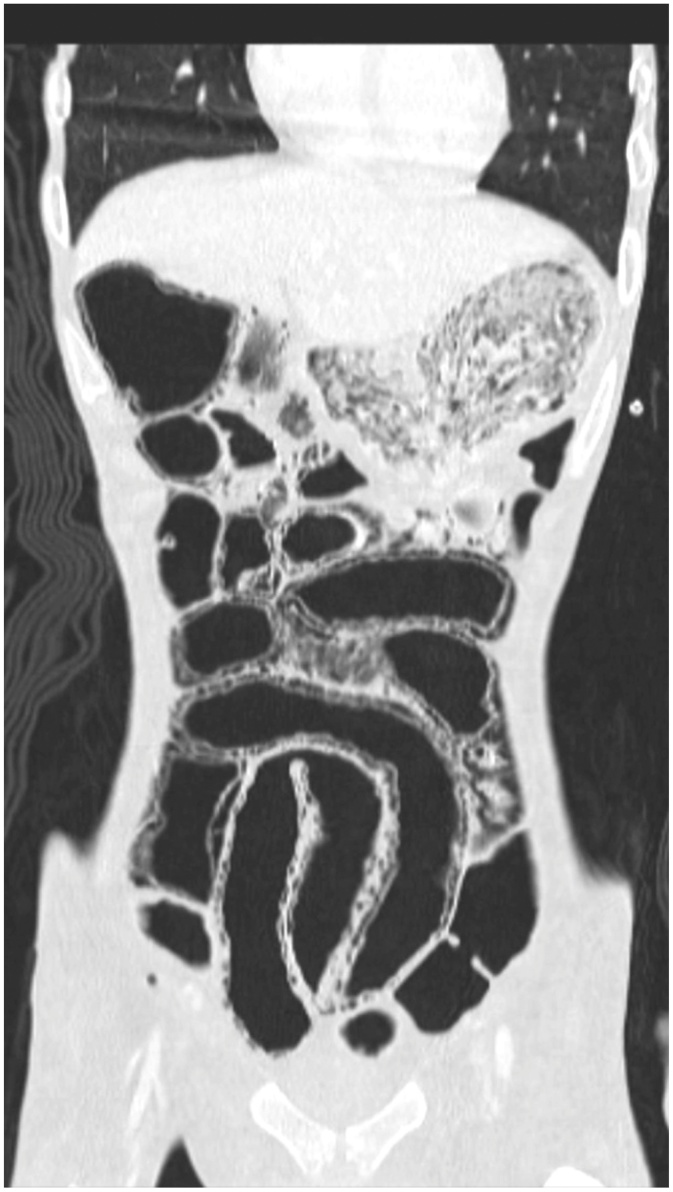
Abdominal coronal computed tomography image. This abdominal reveals extensive pneumatosis, moderate pneumoperitoneum and gas in the venous portal system.
